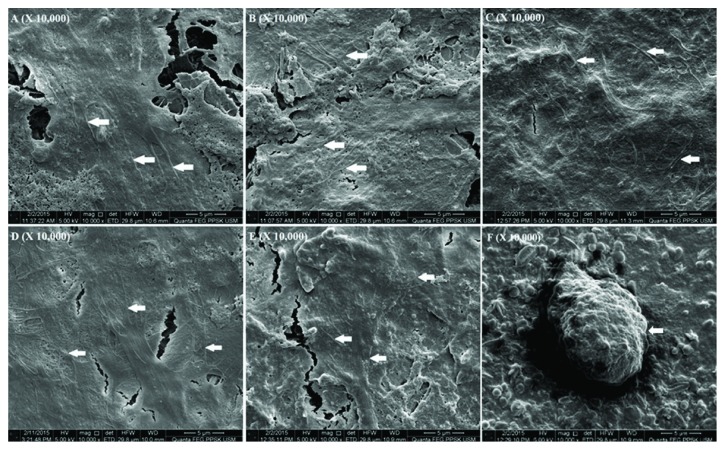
SEM analysis of SHED attachment and growth following 3 days of incubation on (A) Gp-0%CT-BMP material, (B) Gp-1%CT-BMP material, (C) Gp-2.5%CT-BMP material, (D) Gp-5%CT-BMP material, and (E) Gp-10%CT-BMP material. Pictures showing numerous thin cytoplasmic extensions of the cells (white arrows); (F) SEM analysis showing rounded nonliving cell on Dycal (white arrows).
